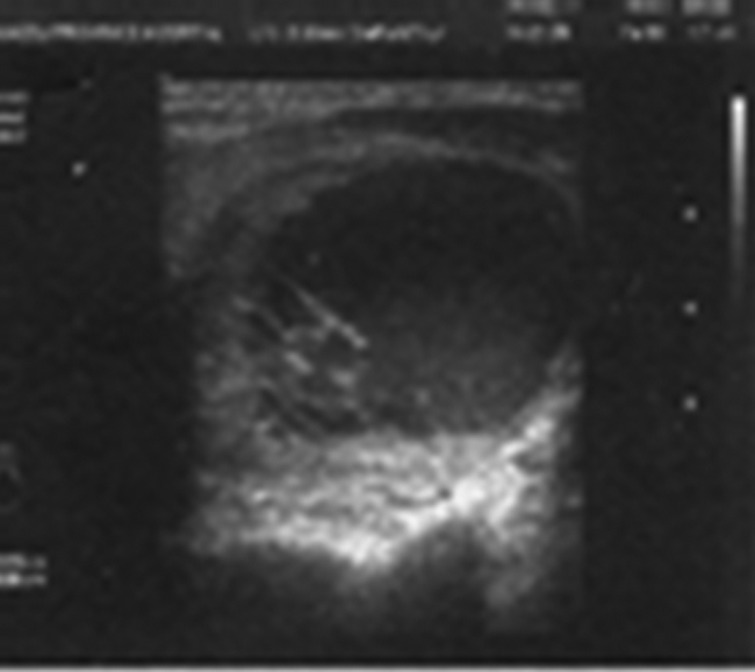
A sagittal ultrasound view demonstrated a 4.03 cm predominant cyst behind the left lobe of the thyroid gland.
